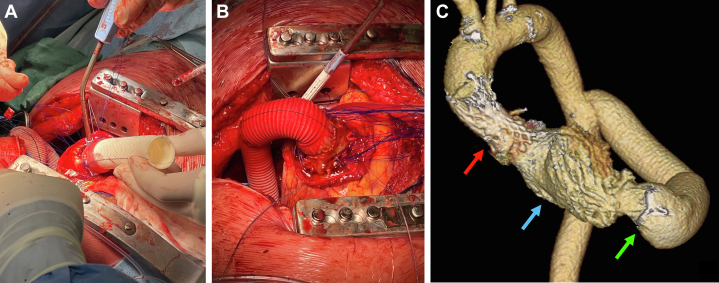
AAC Procedure Surgical beating-heart procedure. An apico-aortic conduit (AAC) including a biological valve prosthesis is sutured to the apex (A) first and then connected to the thoracic aortic graft (B). Post-procedure 3-dimensional computed tomography after AAC between the apex and the thoracic aorta. The red arrow points to the previous self-expandable prosthesis in the heavily calcified hypoplastic aortic root; the blue arrow points to contrast medium in the left ventricle; the green arrow shows the surgical bioprosthetic stent sutured at the beginning of the conduit to ensure 1-way flow and prevent regurgitation (C).
